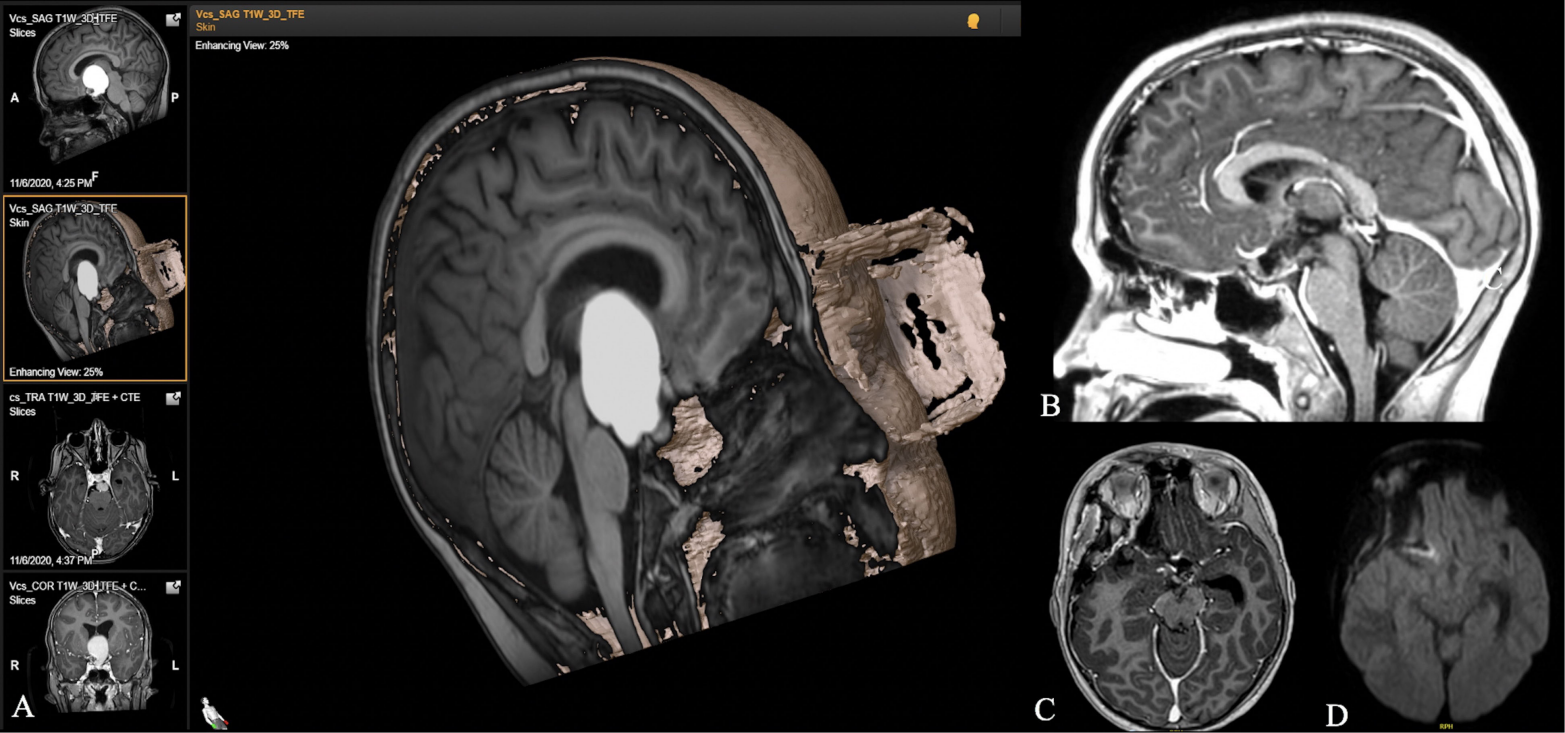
(A) 3D coronal T1-weighted reconstruction and axial coronal T1-weighted contrast-enhanced MR images of a 12-year-old boy diagnosed with a large craniopharyngioma. Sagittal (B) and axial (C) T1-weighted, contrast-enhanced and diffusion-weighted (D) intraoperative images after pterional resection, demonstrating a radical excision without complications. Note the integrity of the pituitary stalk and both the hypothalamic and mammillary bodies.
